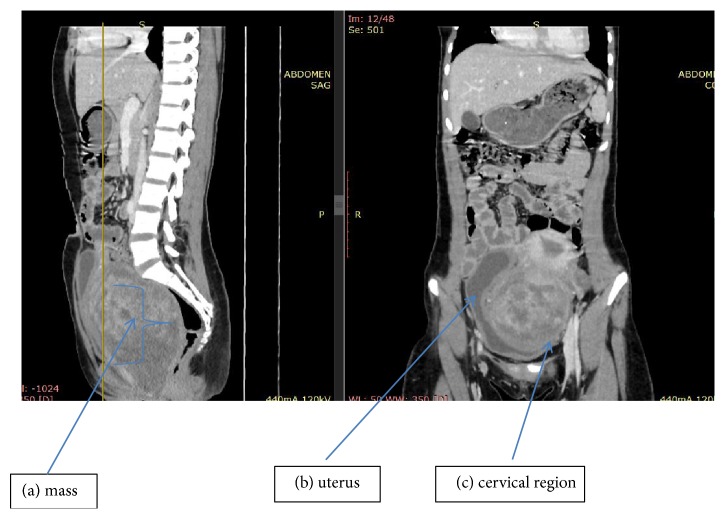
Computed Tomography (CT) scan of the abdomen and pelvis. Arrow heads show hypodense contrast enhancing mass (a) seen on the uterus (b) arising from the cervical region (c).
